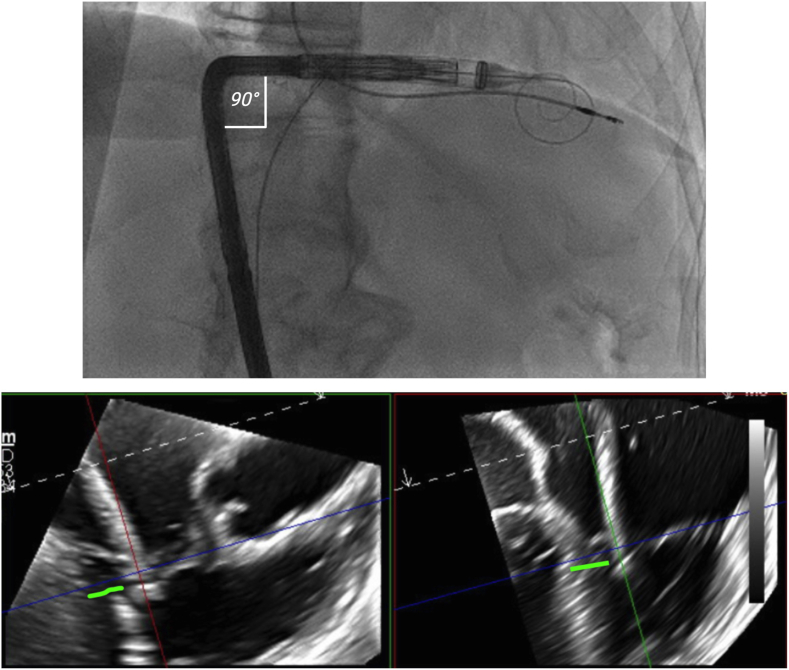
Intraprocedure fluoroscopy and TEE image of the EVOQUE catheter showing closely matching the CT overlay in Figure 2 highlighting the height gain while increasing the need for primary flex on the catheter. The green line indicates the capsule gap that positioned in between the systolic and diastolic leaflet excursion. Abbreviation: TEE, transesophageal echocardiography.
